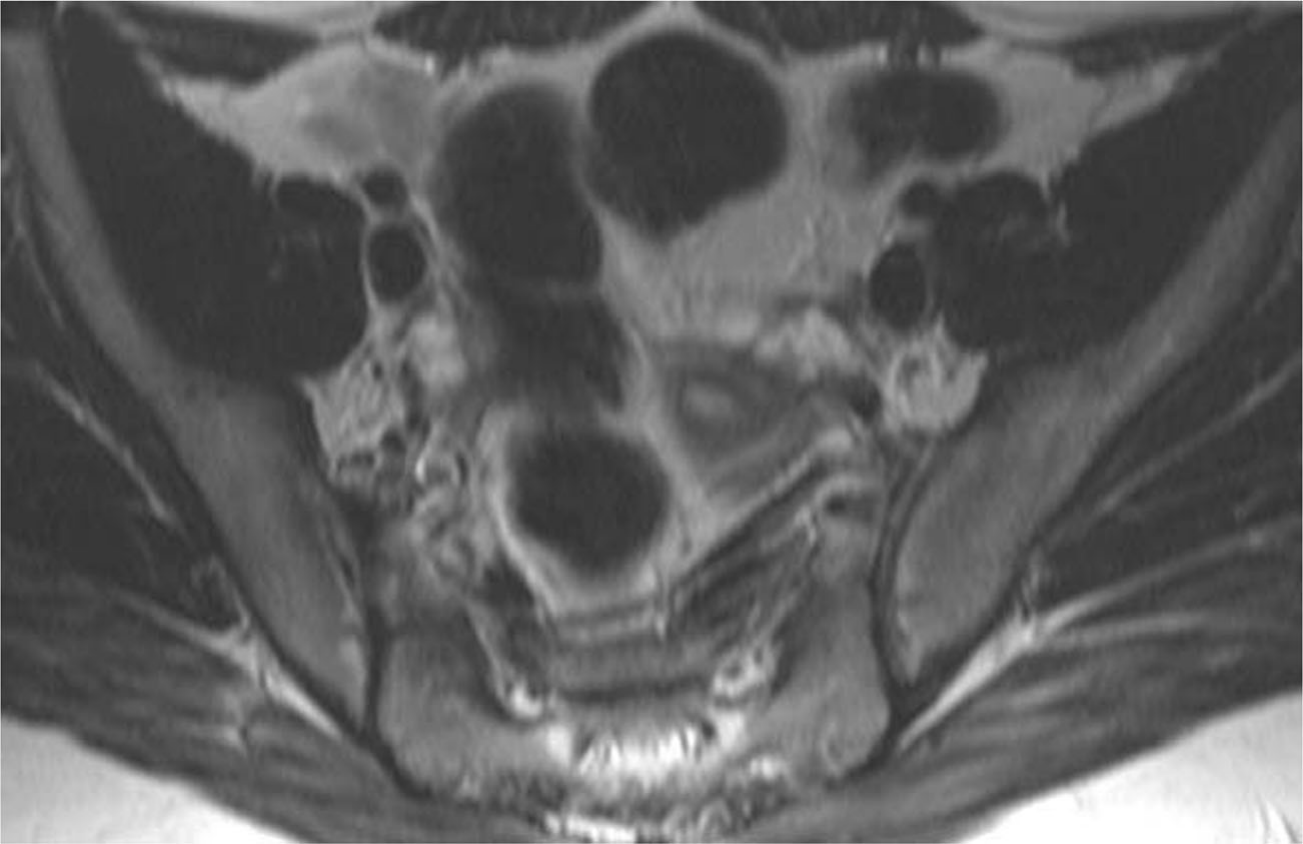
MRI image (no pathology)
